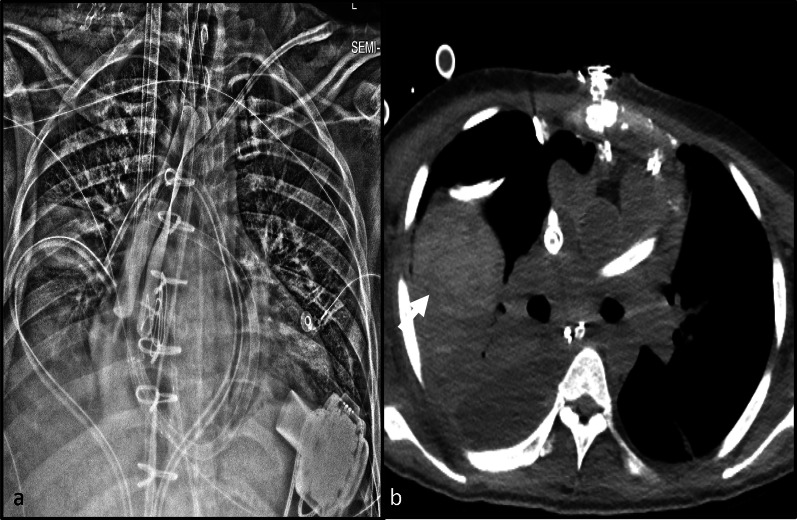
Protek Duo for right ventricle support. AP Chest radiograph with CLAHE filter after Protek Duo placement (a) demonstrating right basilar and mid-lung opacities. The outlet cannula portion should be in right ventricle and the inner portion within the pulmonary artery. In this case, the outlet cannula tip is within the right pulmonary artery. This can cause asymmetric perfusion and edema. Axial CT (b) in mediastinal window demonstrates asymmetric pulmonary opacities, right pleural effusion and a hemothorax (white arrow). (CLAHE—contrast limited adaptive histogram equalization)
